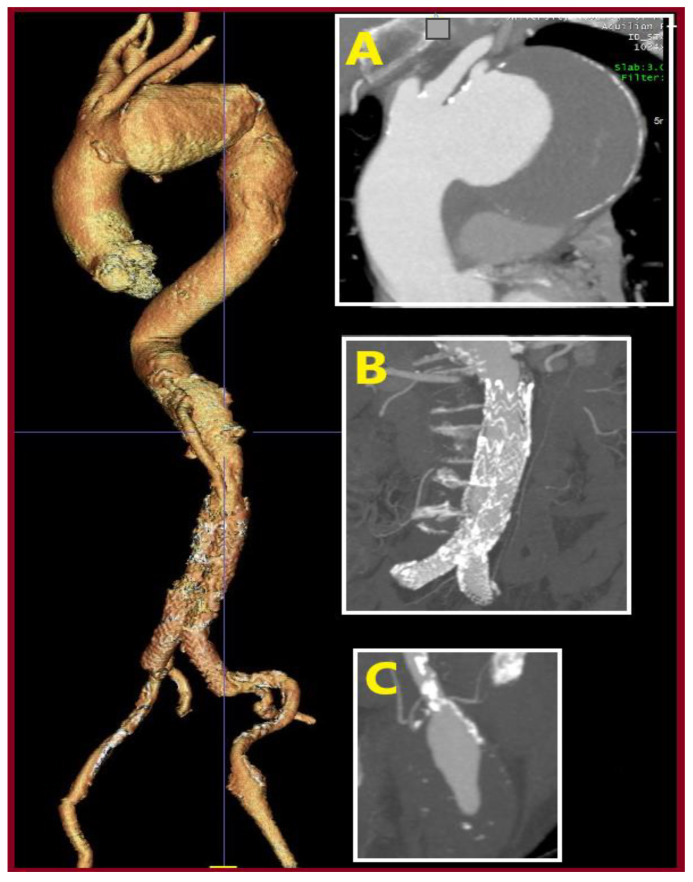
Computed Tomography Angiography (CTA, 3-D Reconstruction) depicting a totally shrunken aortic sac without endoleak, 10 years postoperatively. The graft at the right groin is patent. Unfortunately, two new aneurysms have arisen. (A): A newly diagnosed thoracic aortic aneurysm; (B): the old endograft; (C): a newly diagnosed left common femoral artery aneurysm).
